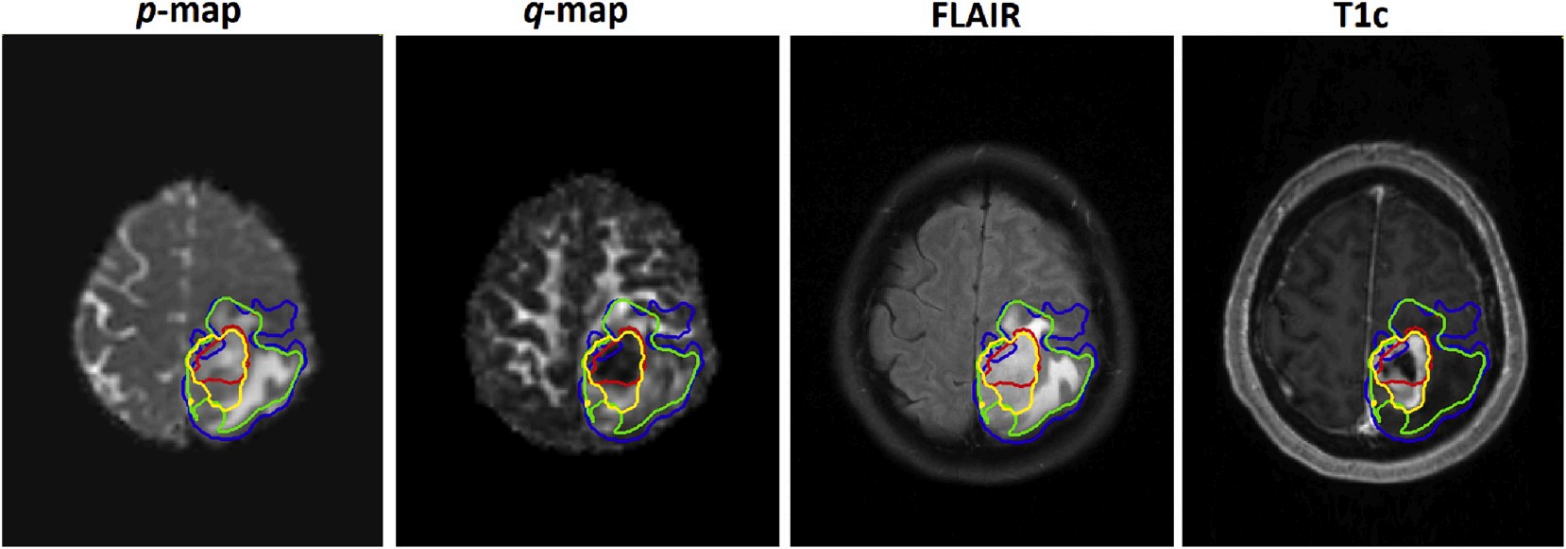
Demonstrating all four segmentations obtained from different models (Models 5–8), where the segmentation in blue is for *p*, red (DC = 80%) for *q*, green (DC = 85%) for FLAIR (DC = 89%) and yellow for T1c (DC = 80%). (For interpretation of the references to colour in this figure legend, the reader is referred to the Web version of this article.)
